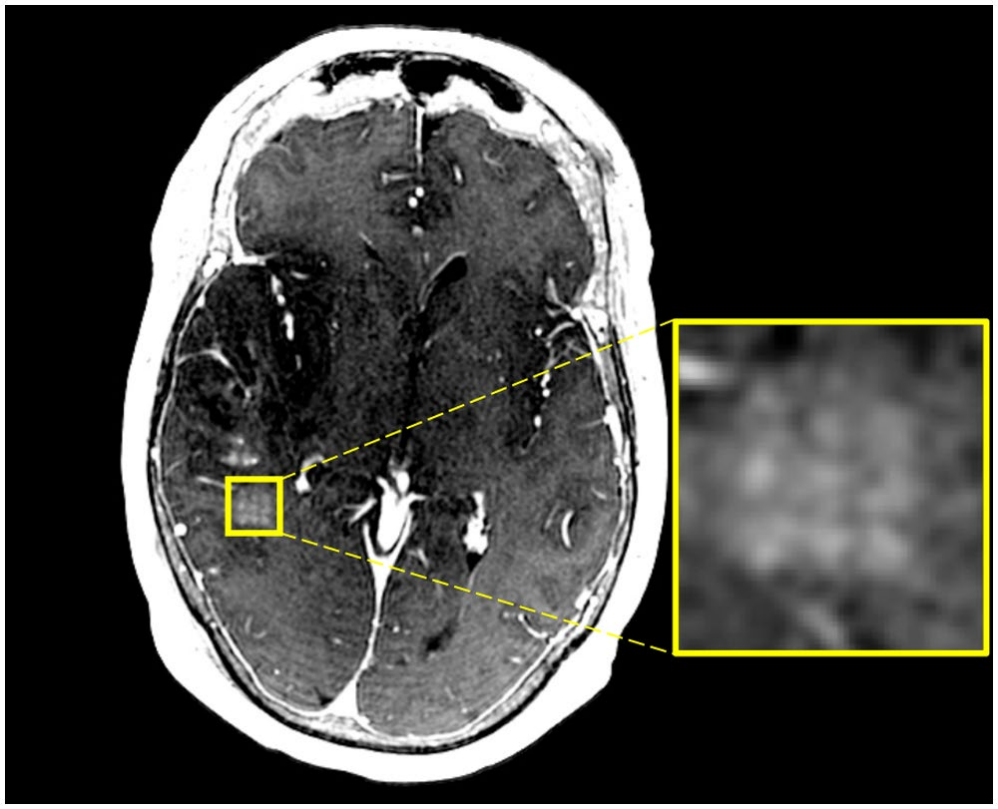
Axial T1-weighted post gadolinium MRI of patient one immediately after MRgFUS BBB disruption demonstrates contrast extravasation in the grid pattern (see enlargement) where sonication occurred. The contrast extravasation is discrete and precise. There is no evidence of edema secondary to the procedure.
